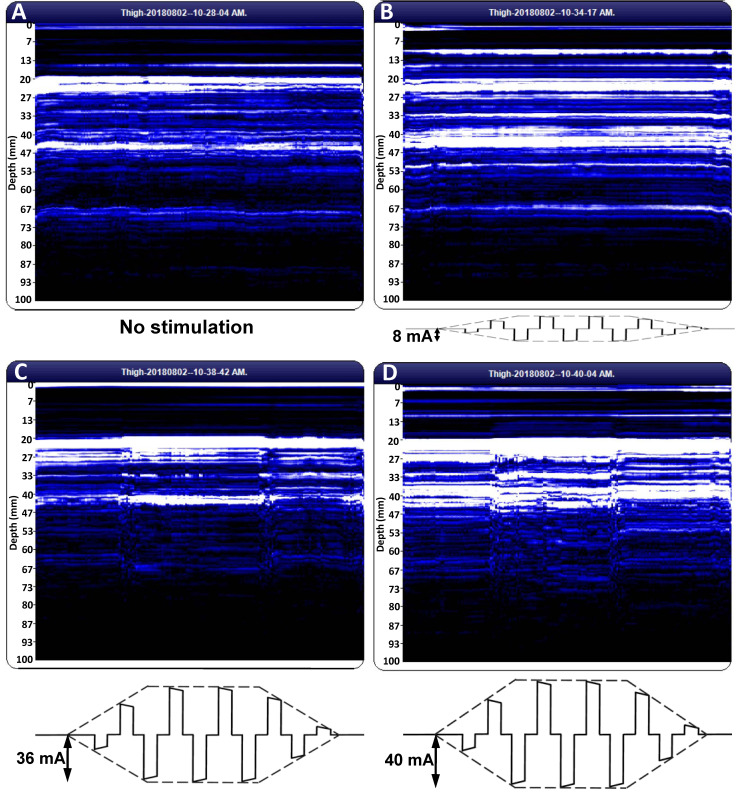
M-mode ultrasound (US) images acquired during neuromuscular electrical stimulation (NMES). US scans of the quadriceps muscle group recorded in the absence (A) and in the presence (B–D) of NMES, with the amplitude of the rectangular pulses set to the sensory threshold (ST) (B), the motor threshold (MT) (C), and 10% above the MT (D). During the scan, the US transducer was maintained in a steady position, midway between the NMES electrodes.
